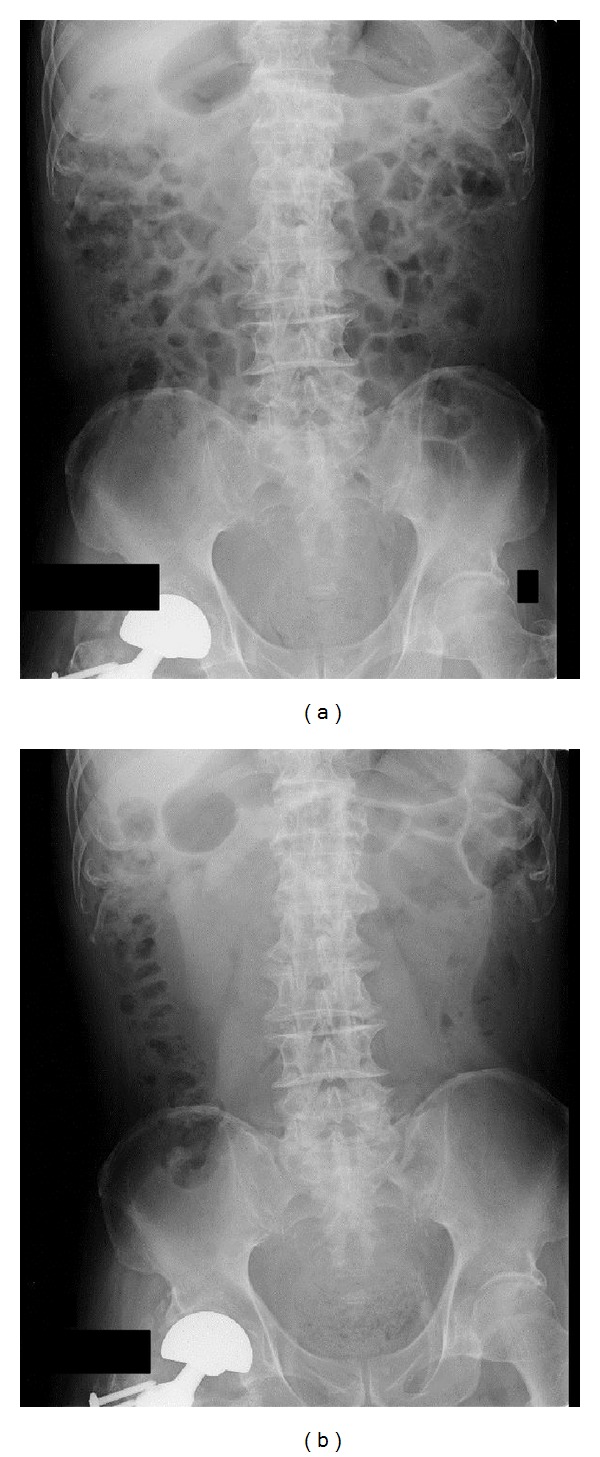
(a) Plain abdominal radiograph of an 86-year-old man prior to Daikenchuto administration. The gas volume score (GVS) was calculated as 26.0%. (b) Plain abdominal radiograph of an 86-year-old man after 4 weeks of Daikenchuto administration. The gas volume score (GVS) was calculated as 12.3%.
